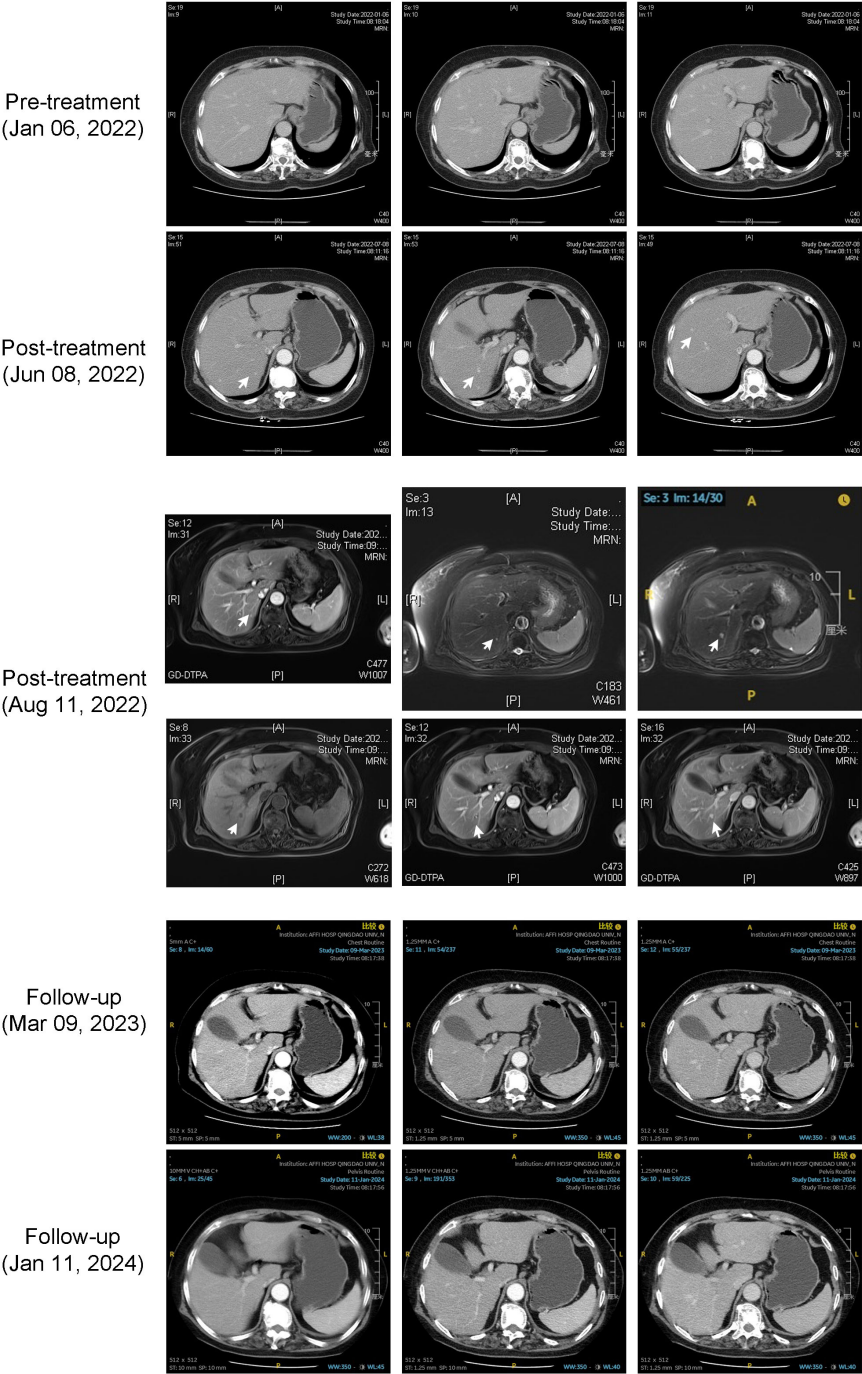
Case 3 hepatic cavernous hemangioma imaging. Combined enhanced CT and MRI manifestations of hepatic cavernous hemangioma in the third case.
